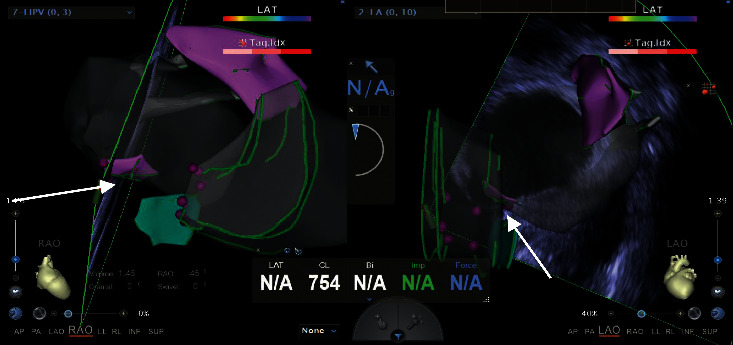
Establishing the left atrium model with intracardiac echocardiography (ICE) and using electroanatomical mapping systems (EAMs) and ICE to confirm the location of the foramen ovale (shown by the arrow).
